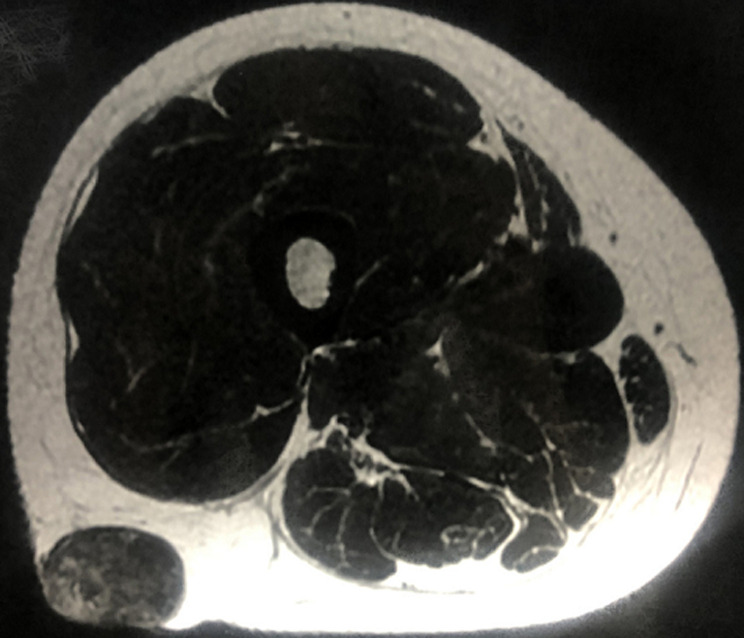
magnetic resonance imaging showing very limited and homogeneous subcutaneous calcification
